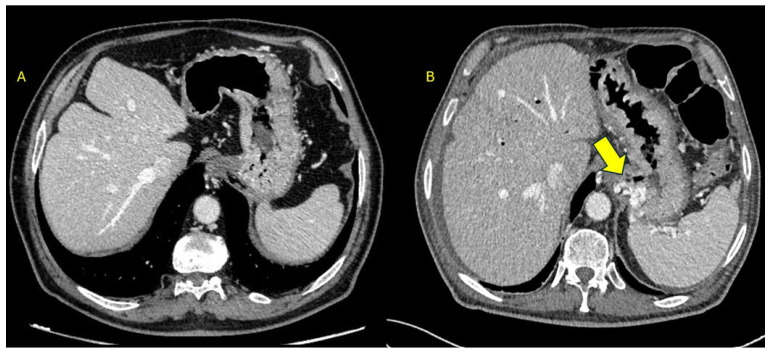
Sagittal imaging. Comparison between pre-operative (A) and post-operative (B) CT scans. The arrow indicates the iatrogenic portosystemic shunt between the portal territory and the azygous vein.
